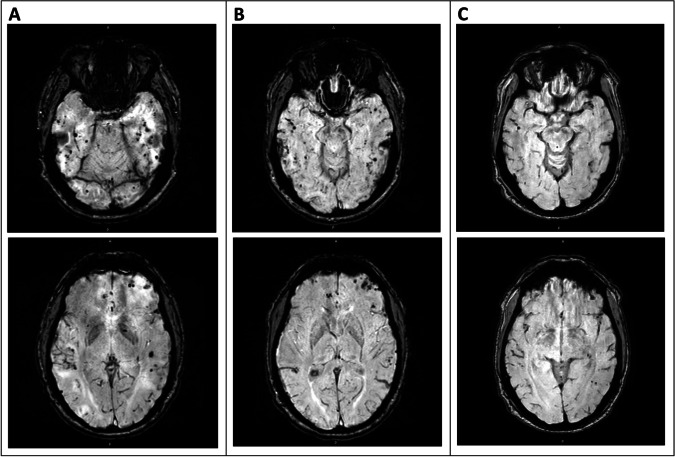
SWI MRI sequences both high and low cuts (A) Initial Presentation (B) 1-month after stopping Mepolizumab C) 6-months after stopping Mepolizumab
